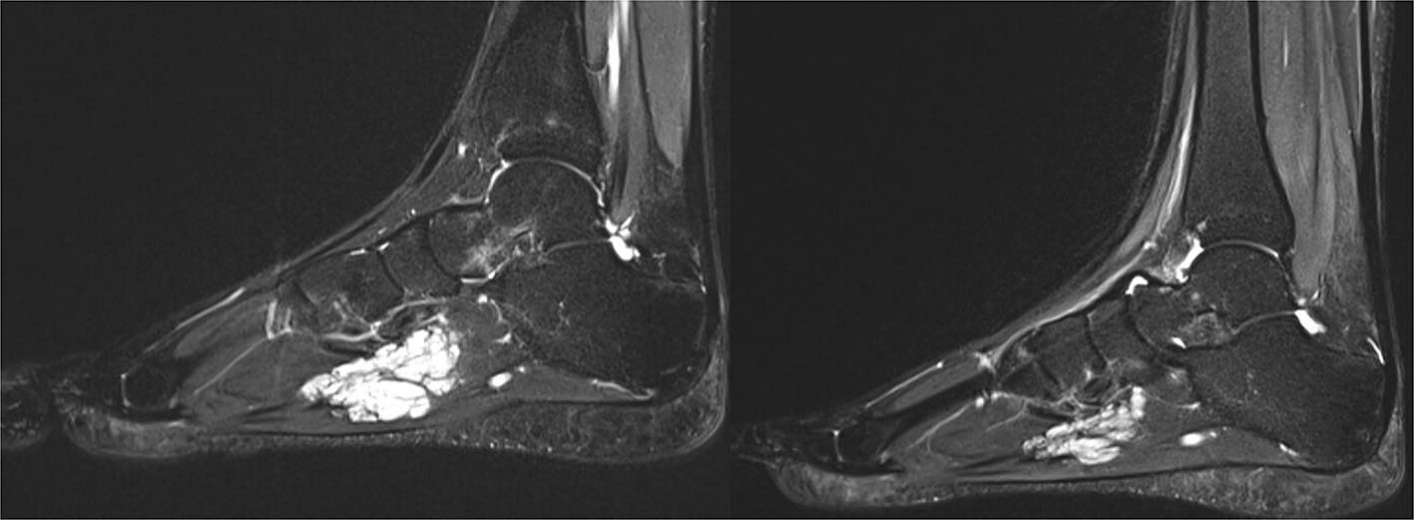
Patient number 8. MRI (T2 STIR sagit. plane) of the right foot prior (left) and 3 months after alpelisib treatment (right).
